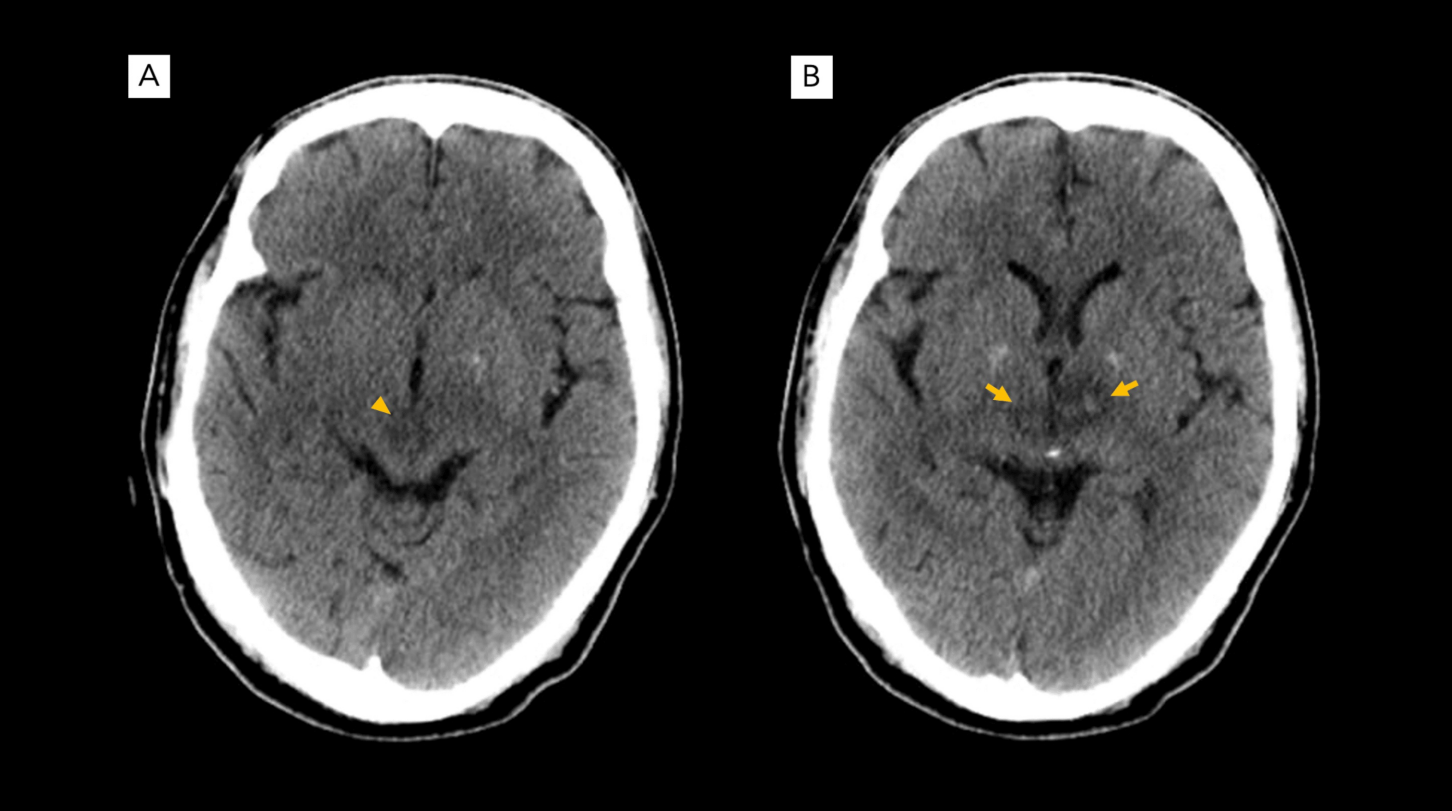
Cranial CT scan findings Non-contrast enhanced cranial CT scan taken eight hours post-ictus reveals an acute hemorrhagic infarction of the rostral mesencephalon (A, arrowhead) and bilateral thalami (B, arrows).
